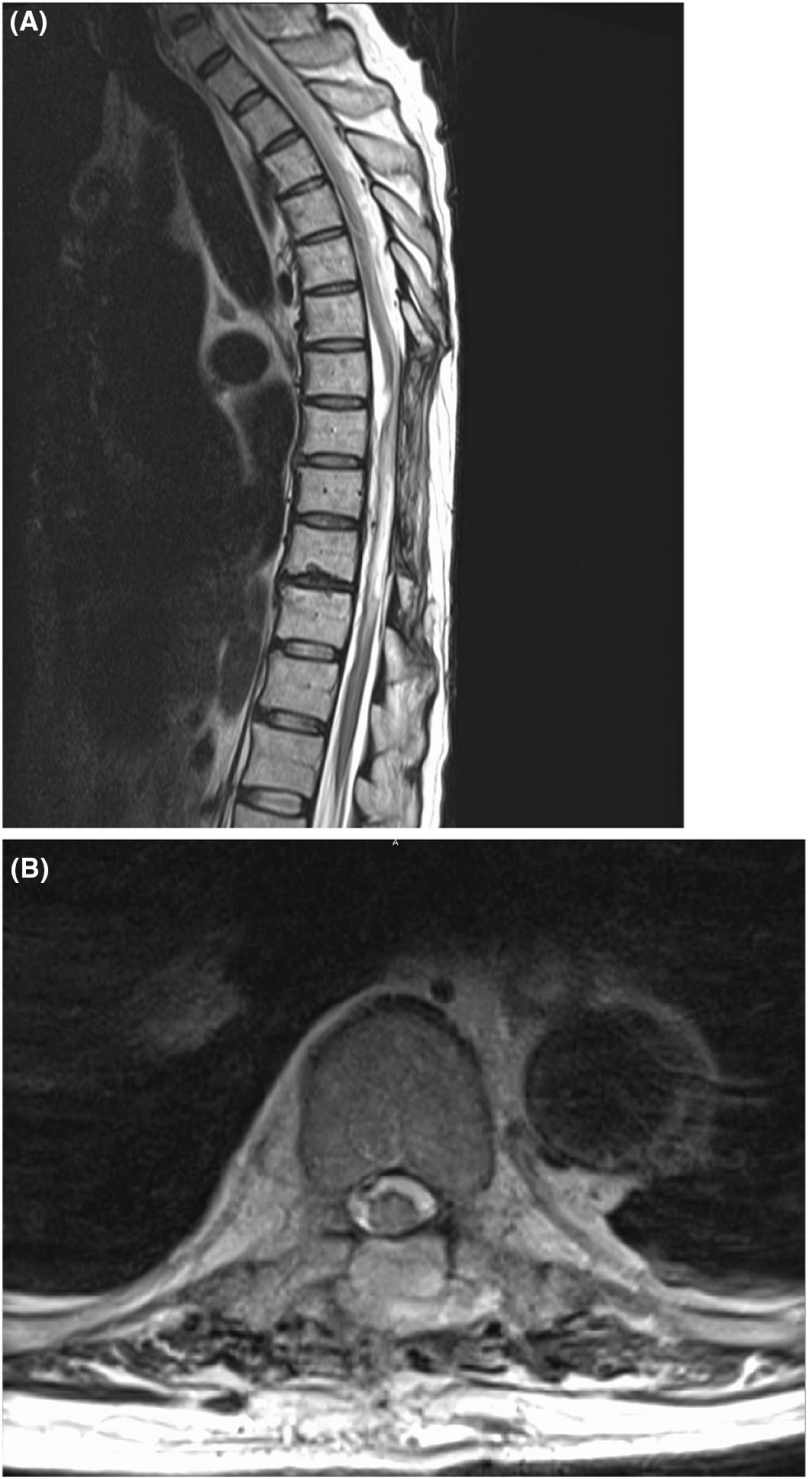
MRI 3 months after surgery. (A) Sagittal T2‐weighted MRI showed cerebrospinal fluid collecting on the ventral side of the spinal cord, with findings of adhesive arachnoiditis following subdural hematoma. (B) Axial T2‐weighted MRI also showed fluid collection on the ventral side.
